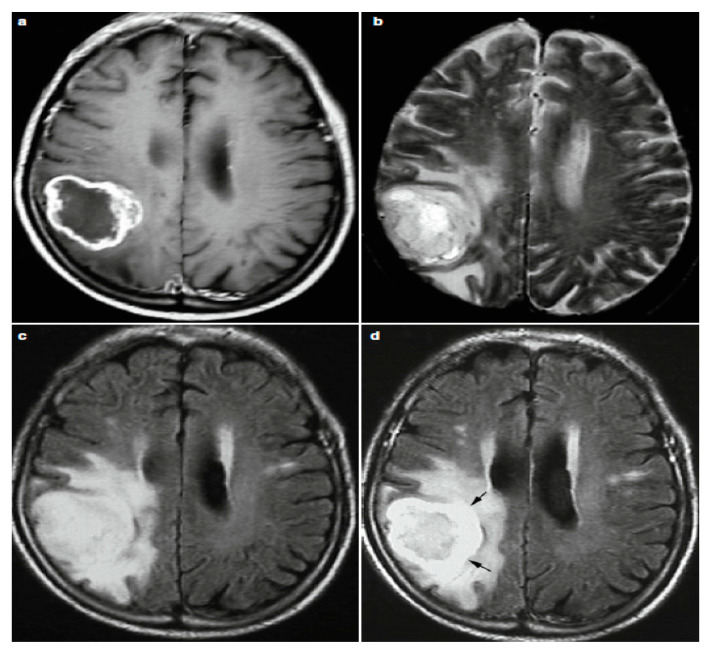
Four Different Image Modalities: (a) Post-Contrast T1w, (b) T2w, (c) FLAIR and (d) Post-Contrast FLAIR MRI.
